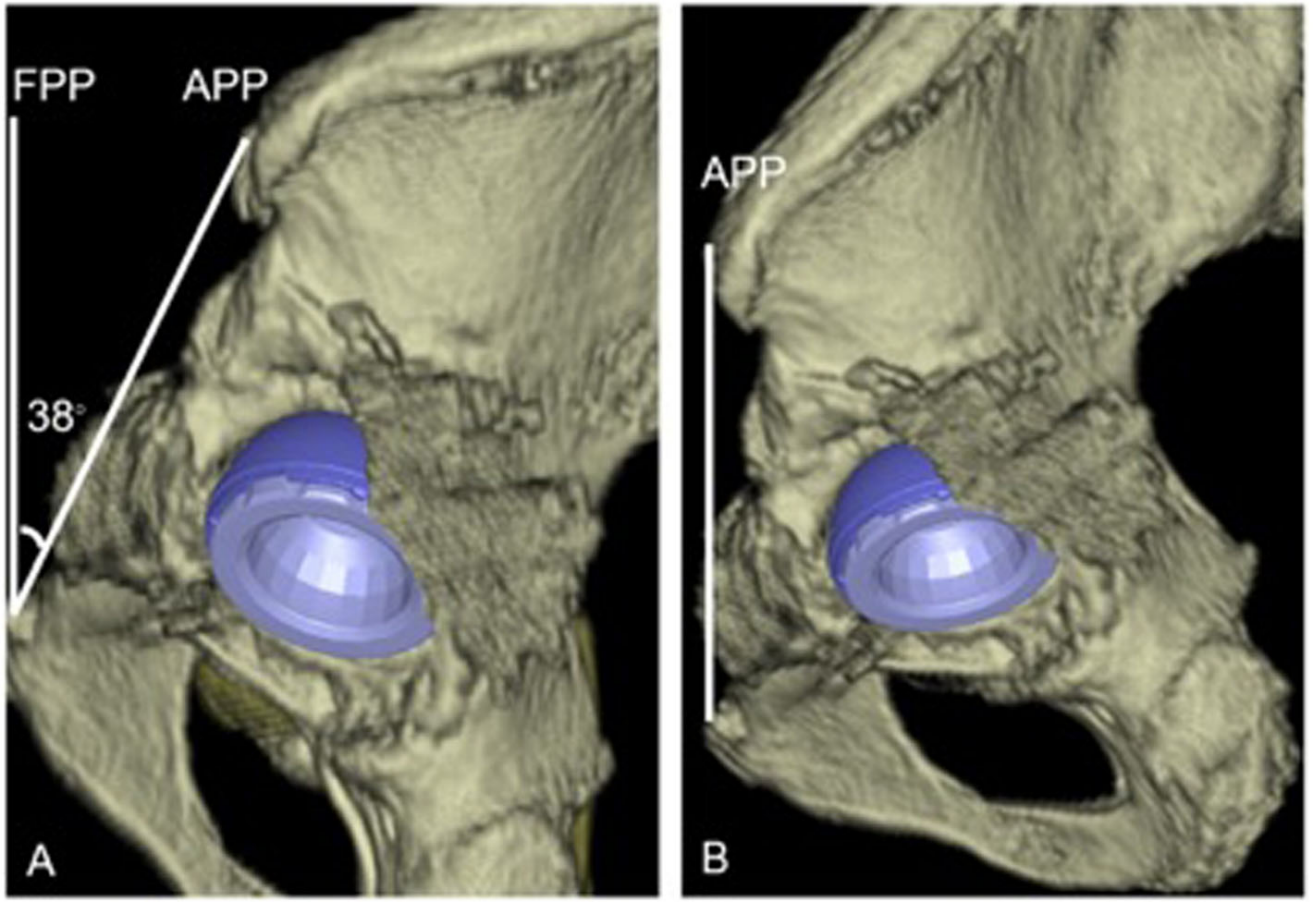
Preoperative planning of cup placement on 3D images. a Lateral view of the pelvis with the cup in the horizontal plane. The APP tilting angle in the supine position on CT images was 38 degrees retroversion for the horizontal plane. Because of stem anteversion, the inclination was planned radiographically to be 40 degrees and the anteversion was planned to be 26 degrees relative to the FPP. b Lateral view of the pelvis with the cup in the APP, showing a cup angle of inclination of 37 degrees and a cup angle of anteversion of − 5 degrees relative to the APP
